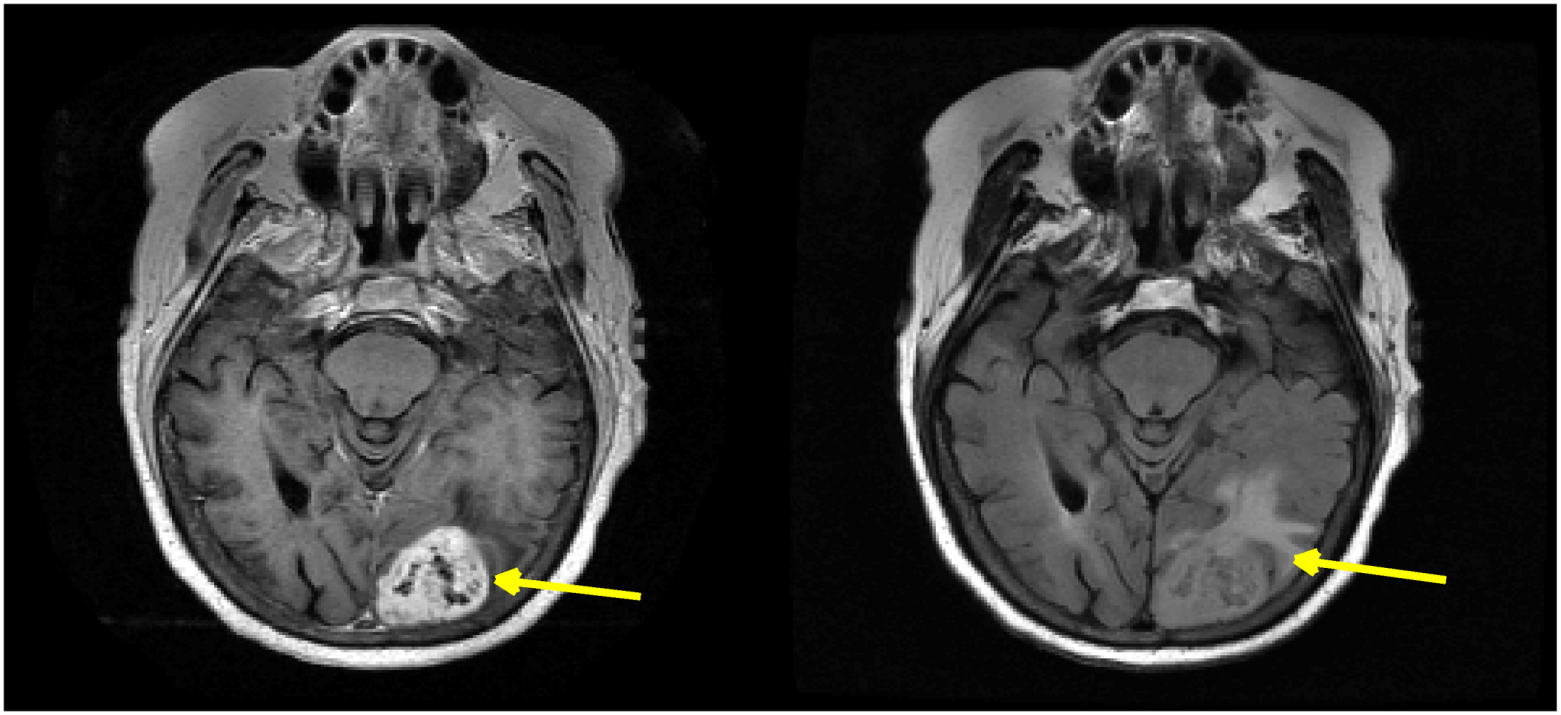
T1 post-contrast image (left) and T2 FLAIR image (right) after preprocessing. Arrows indicate the enhanced tumor area.
